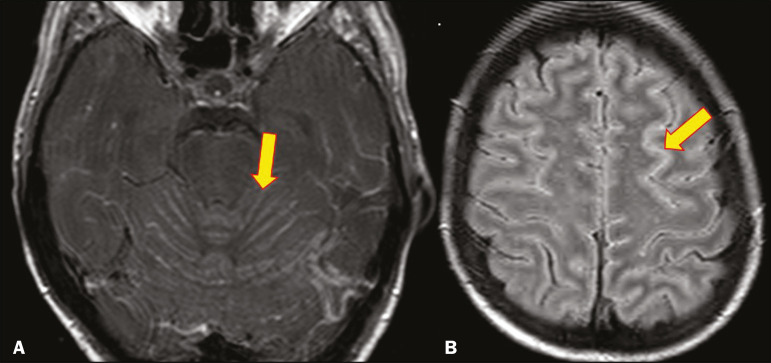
Cryptococcosis meningitis. A: Contrast-enhanced axial T1-weighted MRI sequence showing leptomeningeal enhancement (arrow). B: Axial FLAIR sequence showing leptomeningeal hyperintensity.
